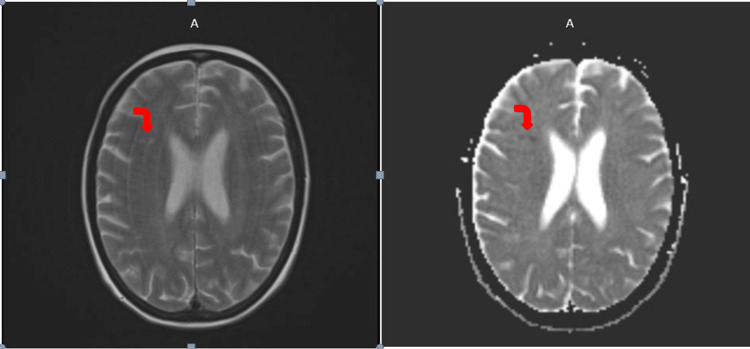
Axial MRI brain (T1- and T2-weighted sequences) showing right insular infarct (red arrows).
